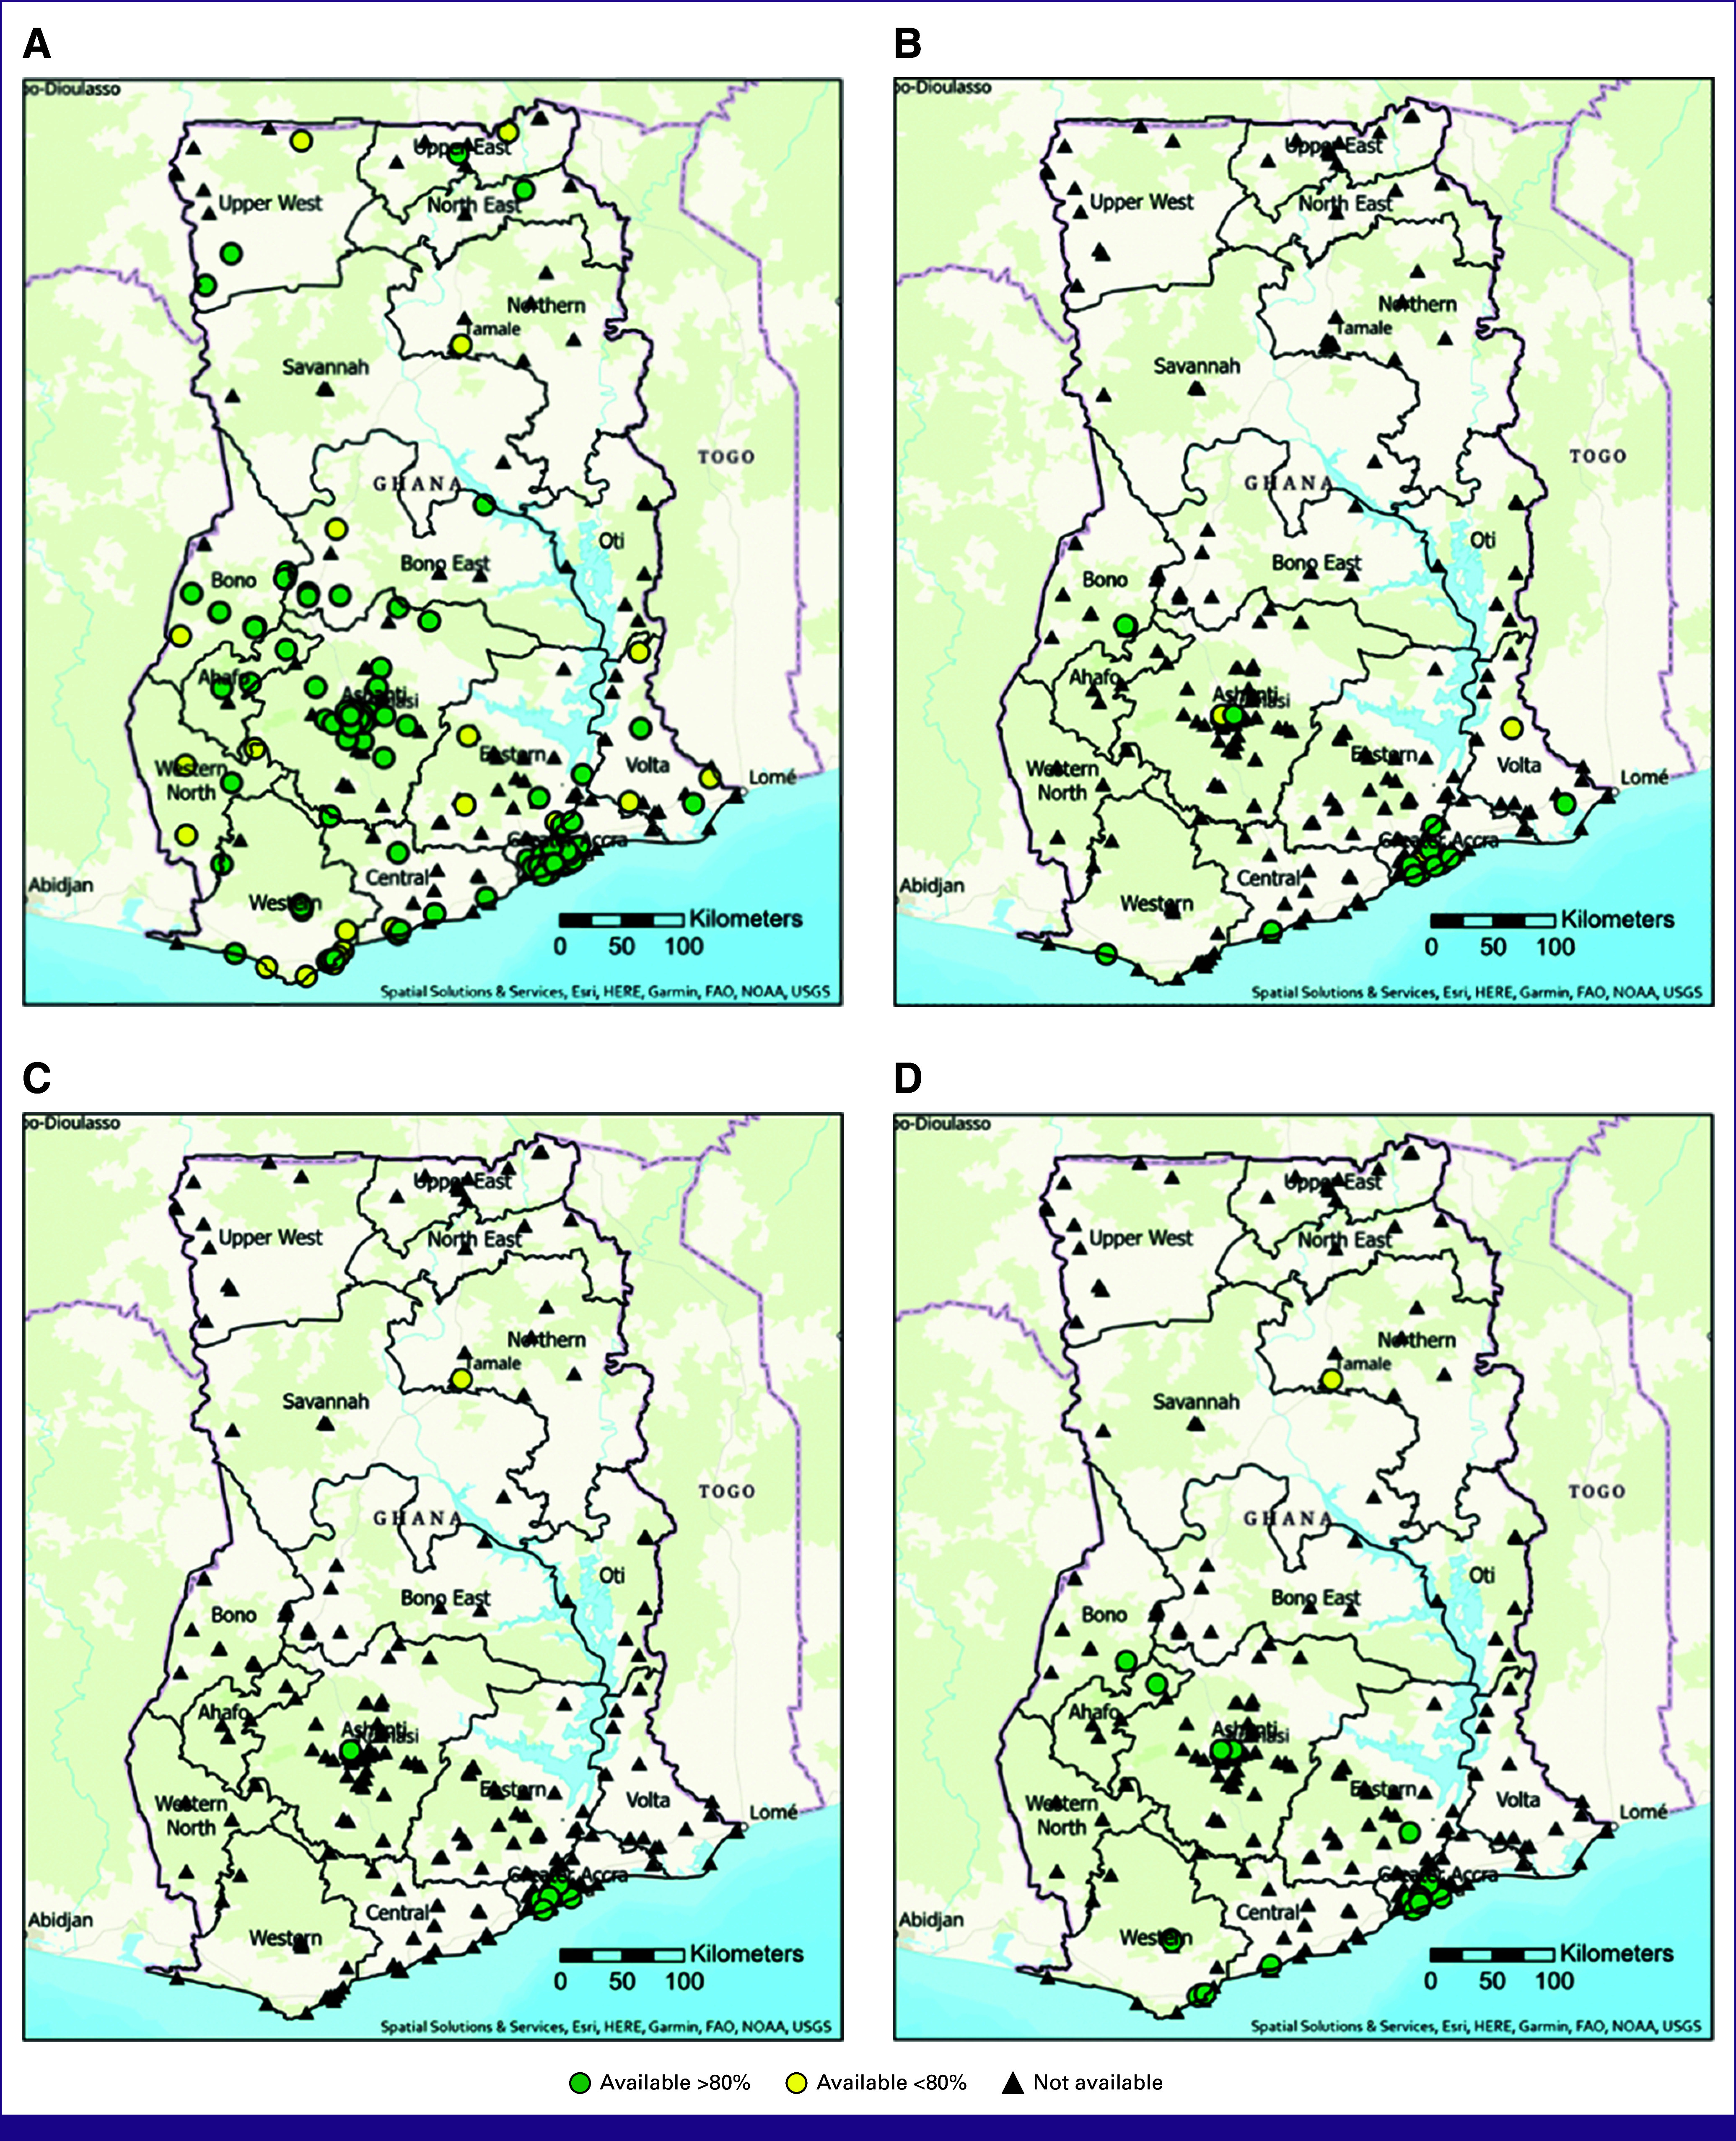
Individual imaging service availability. (A) Diagnostic breast ultrasound; (B) diagnostic mammography; (C) breast MRI; and (D) CT scan. CT, computed tomography; FAO, Food and Agriculture Organization; MRI, magnetic resonance imaging; NOAA, National Oceanic and Atmospheric Administration; USGS, United States Geological Survey.
